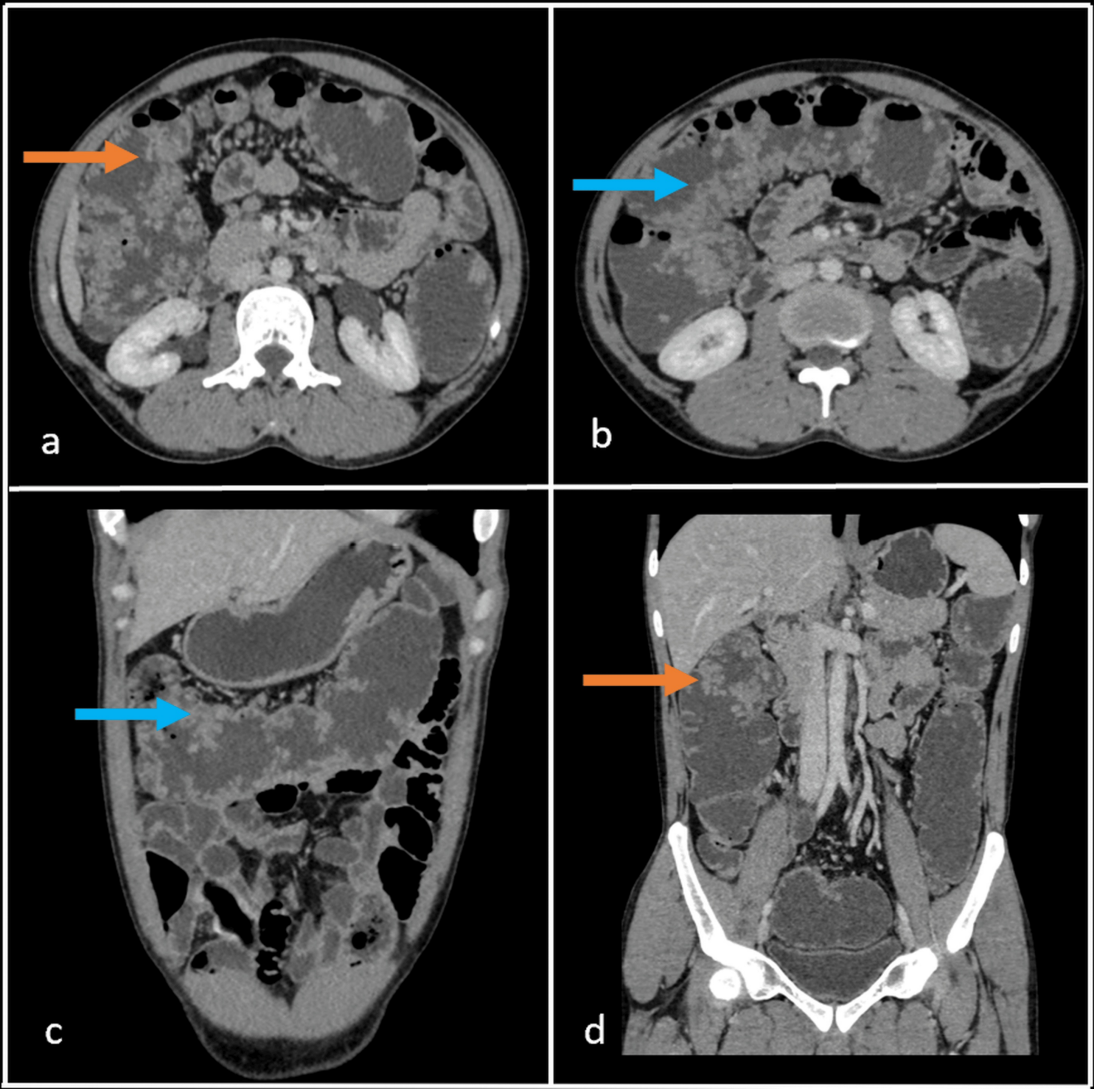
Contrast-enhanced computed tomography (CT) abdomen axial (a, b) and coronal (c, d) sections venous phase showing homogenously enhanced multiple soft tissue polypoidal lesions spanning the entire colon- ascending (orange arrow), transverse, and descending colons, with maximum involvement seen in the transverse colon (blue arrow).
